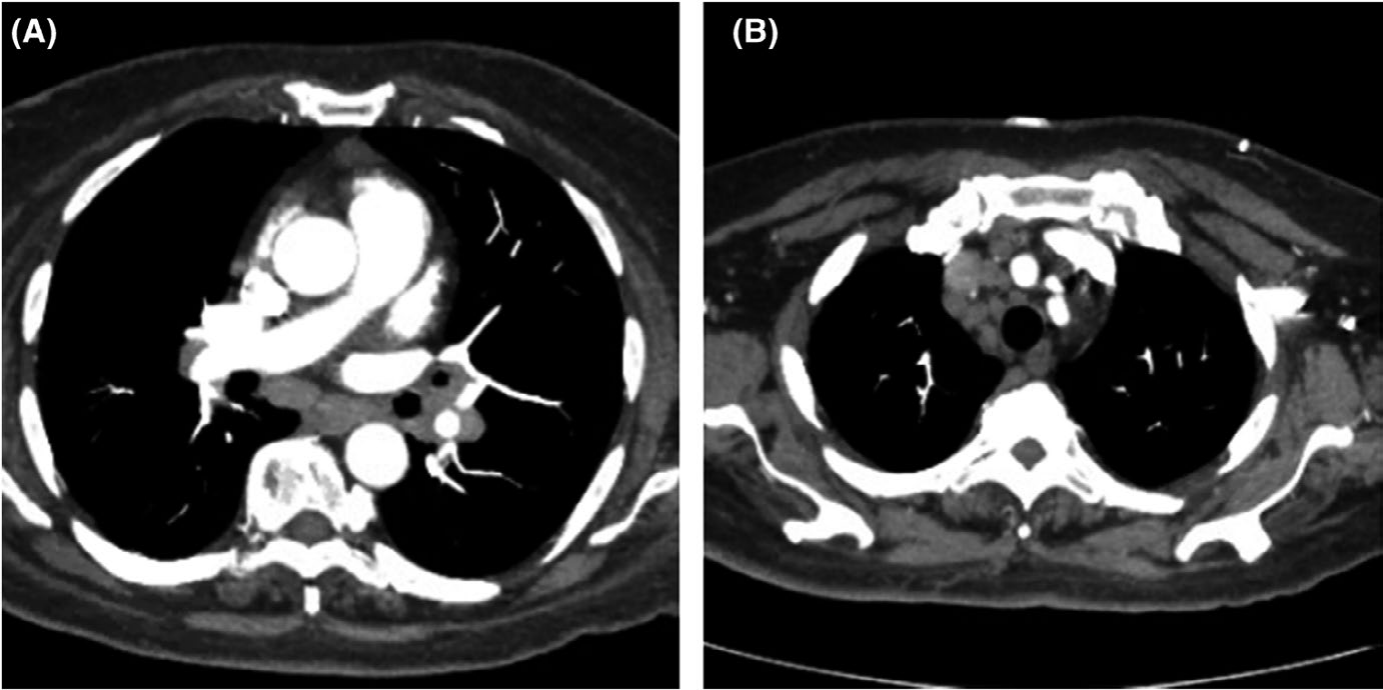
Computed tomography angiography (CT) of the chest showing new‐onset mediastinal (A) and hilar (B) lymphadenopathy
